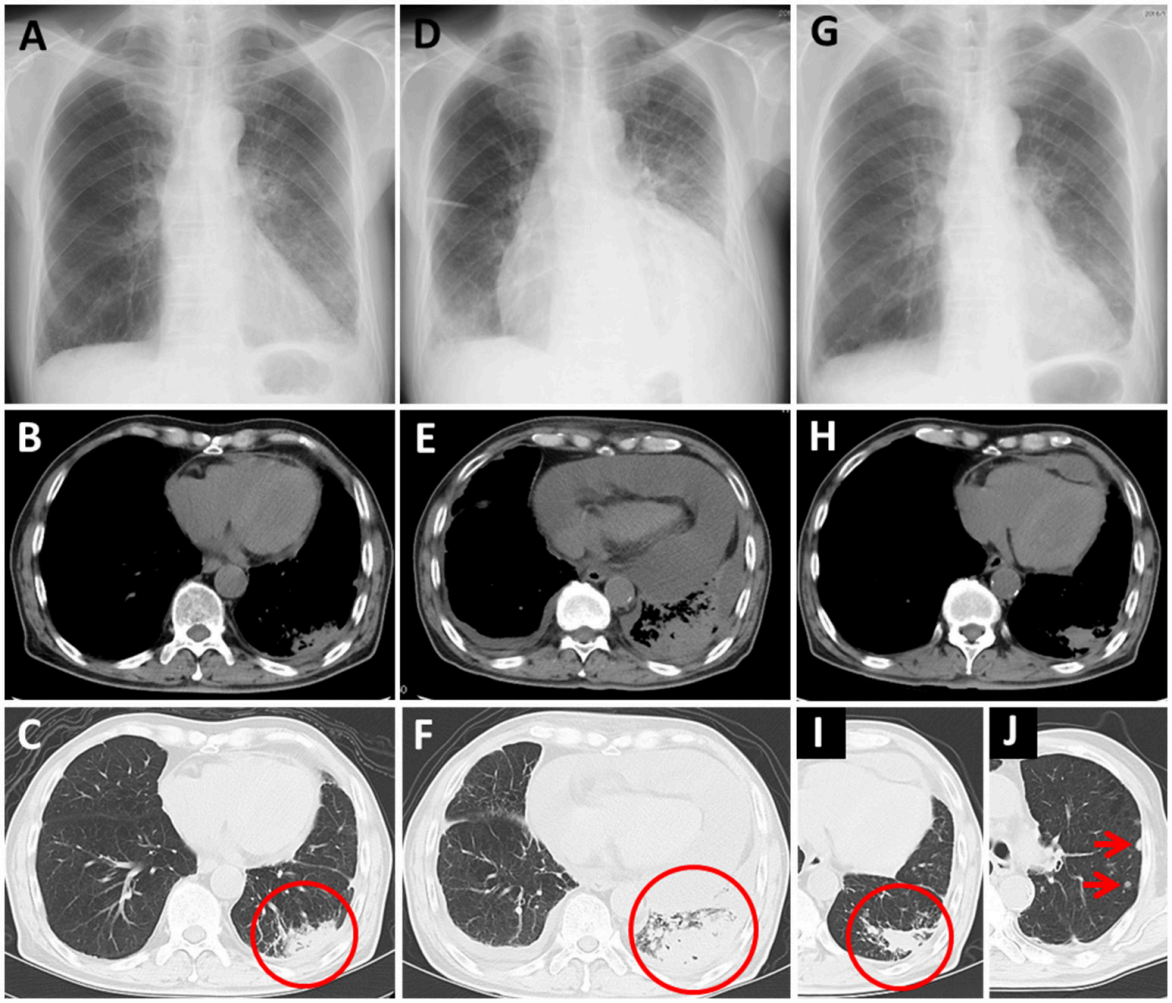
Case 2 (A–C) Chest X-ray and computed tomography (CT) before nivolumab administration. Primary lung cancer lesion (circle) and a small amount of pericardial effusion are detected. (D) Chest X-ray after nivolumab administration shows cardiomegaly. (E,F) Chest CT after nivolumab administration shows massive pericardial effusion and enlargement of the primary lesion (circle). (G) Chest X-ray 2 months after the second pericardiocentesis shows no cardiomegaly. (H–J) Chest CT 2 months after the second pericardiocentesis shows a decrease in pericardial effusion, reduction of the primary lesion (circle), and a few new intrapulmonary lesions (arrows).
